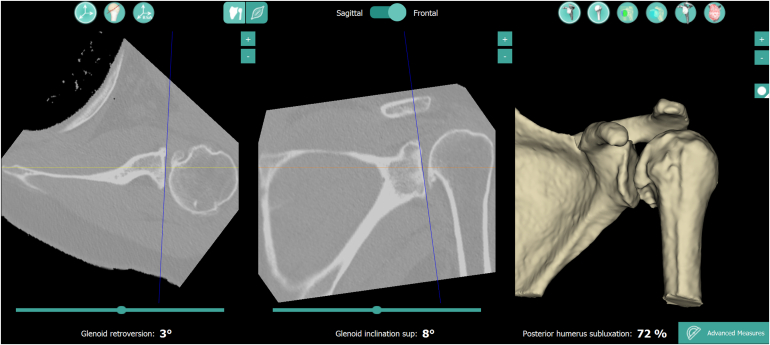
Software interface, calculation of inclination and glenoid version from CT scans data *CT*, computed tomography..
